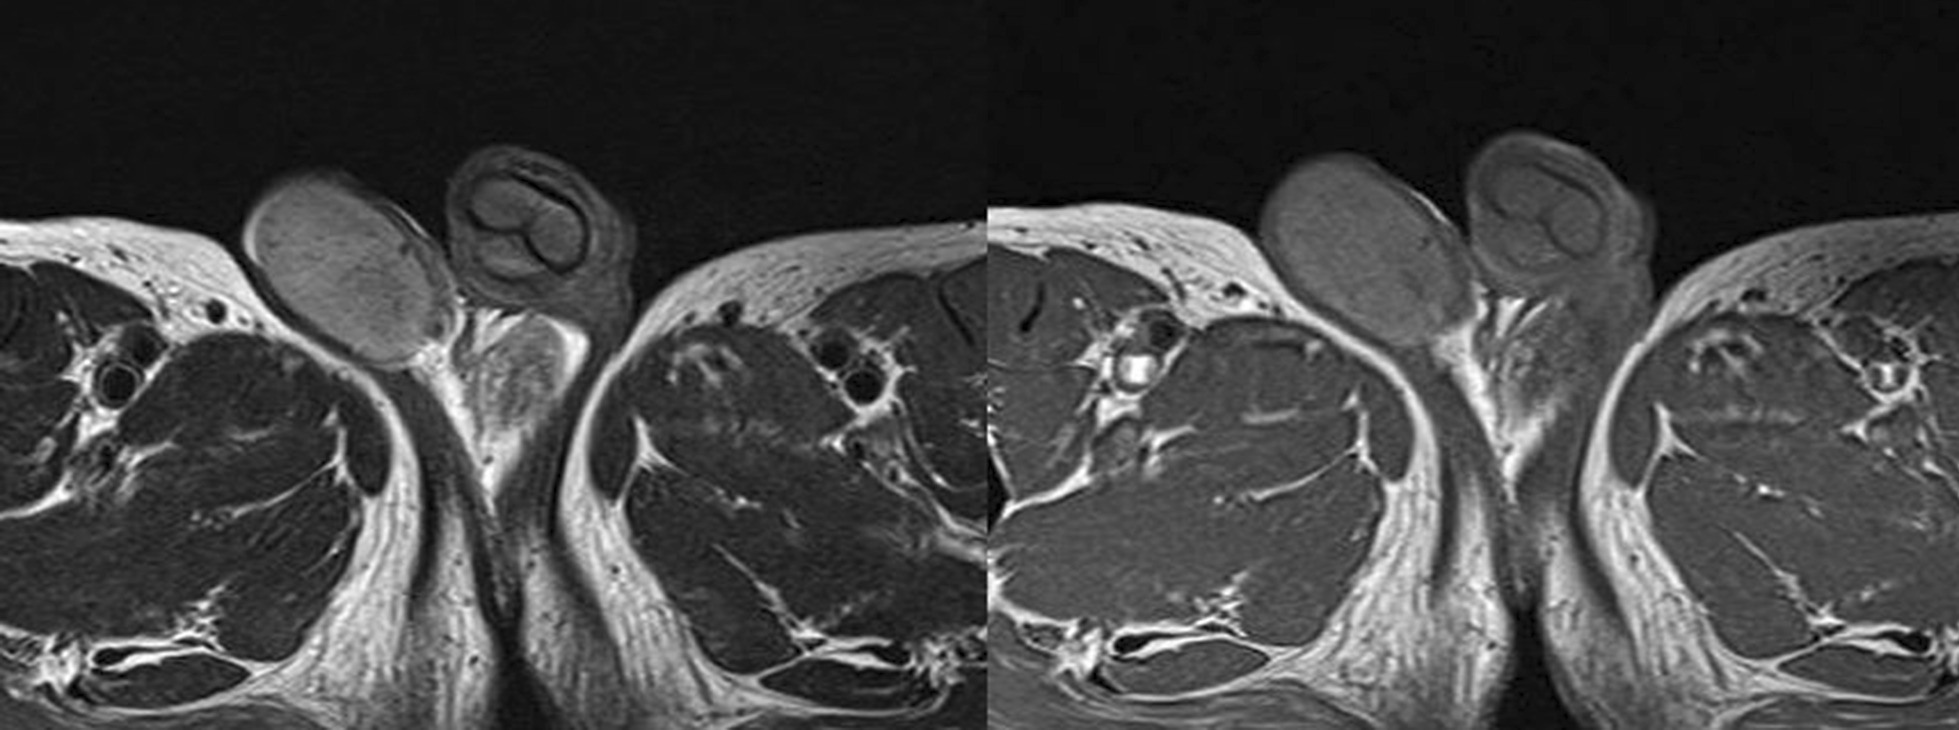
Magnetic resonance imaging of the bilateral testes
